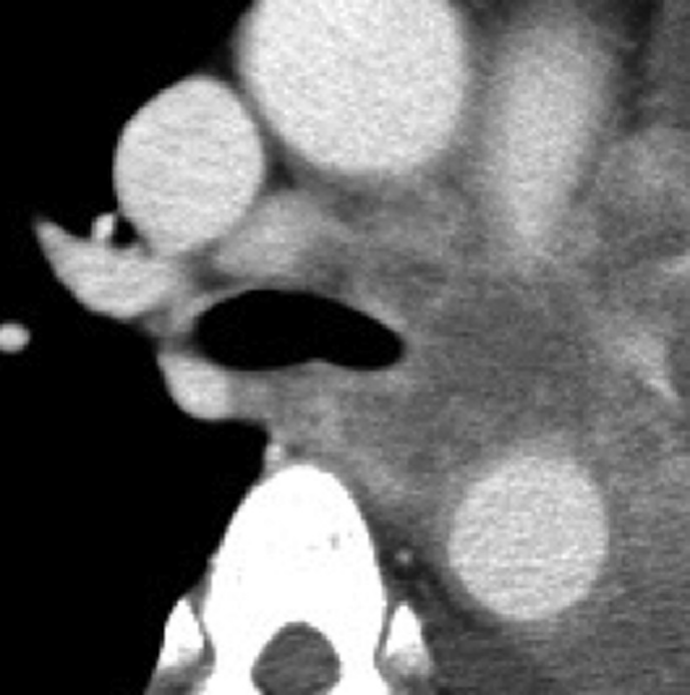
Representative cut of thoracic CT scan showing extranodal invasion of lymph nodes.
